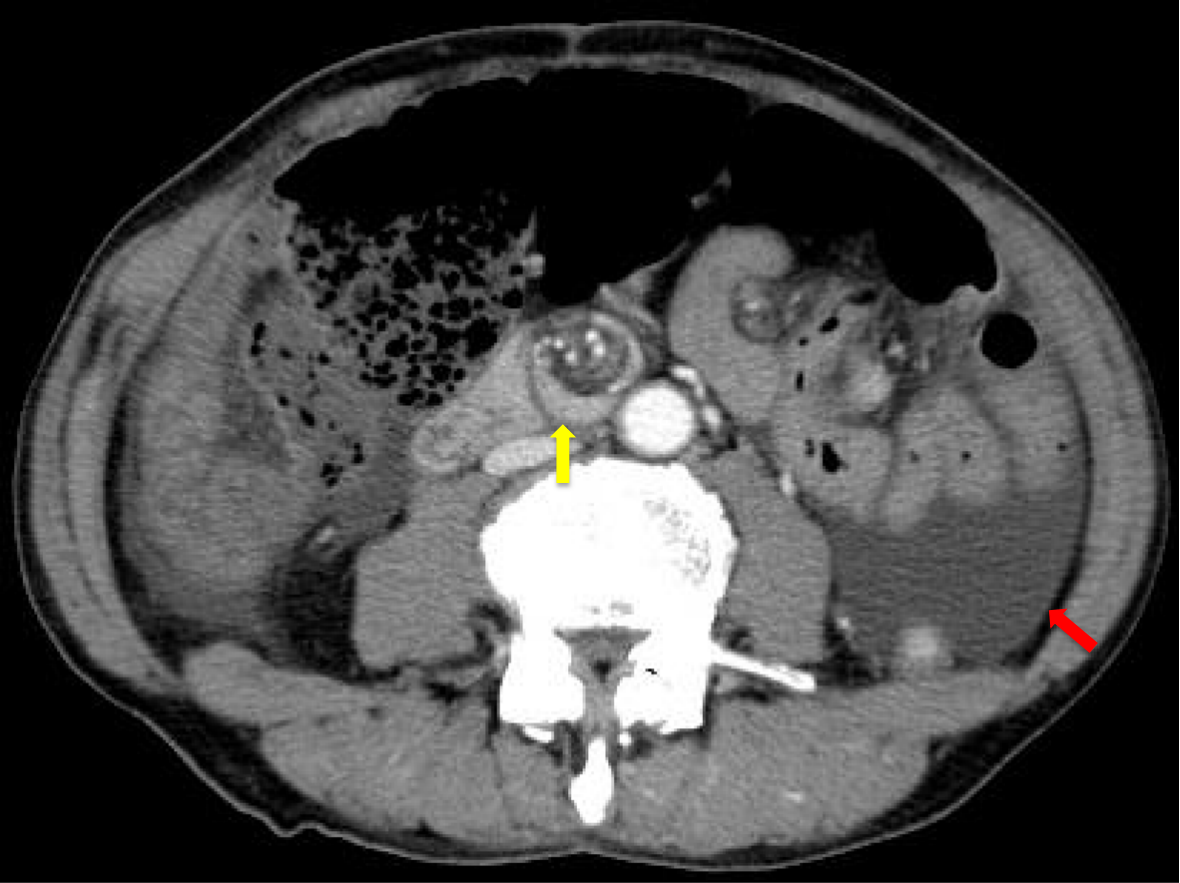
CT scan showing a large amount of ascites (*red arrow*) and whorl-like appearance of the superior mesenteric artery (SMA) and branches with adjacent collapsed loops of small bowel (*yellow arrow*)
